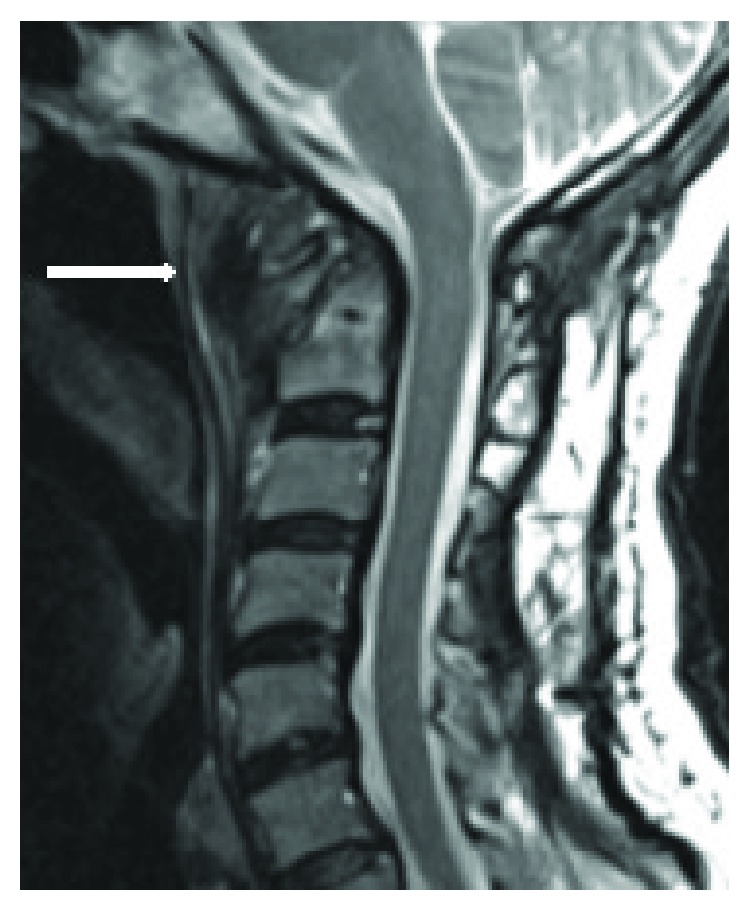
Sagittal T2-weighted image of magnetic resonance imaging of the cervical spine at the first visit showing a mass on retropharyngeal space with heterogeneous intensity (white arrow).
